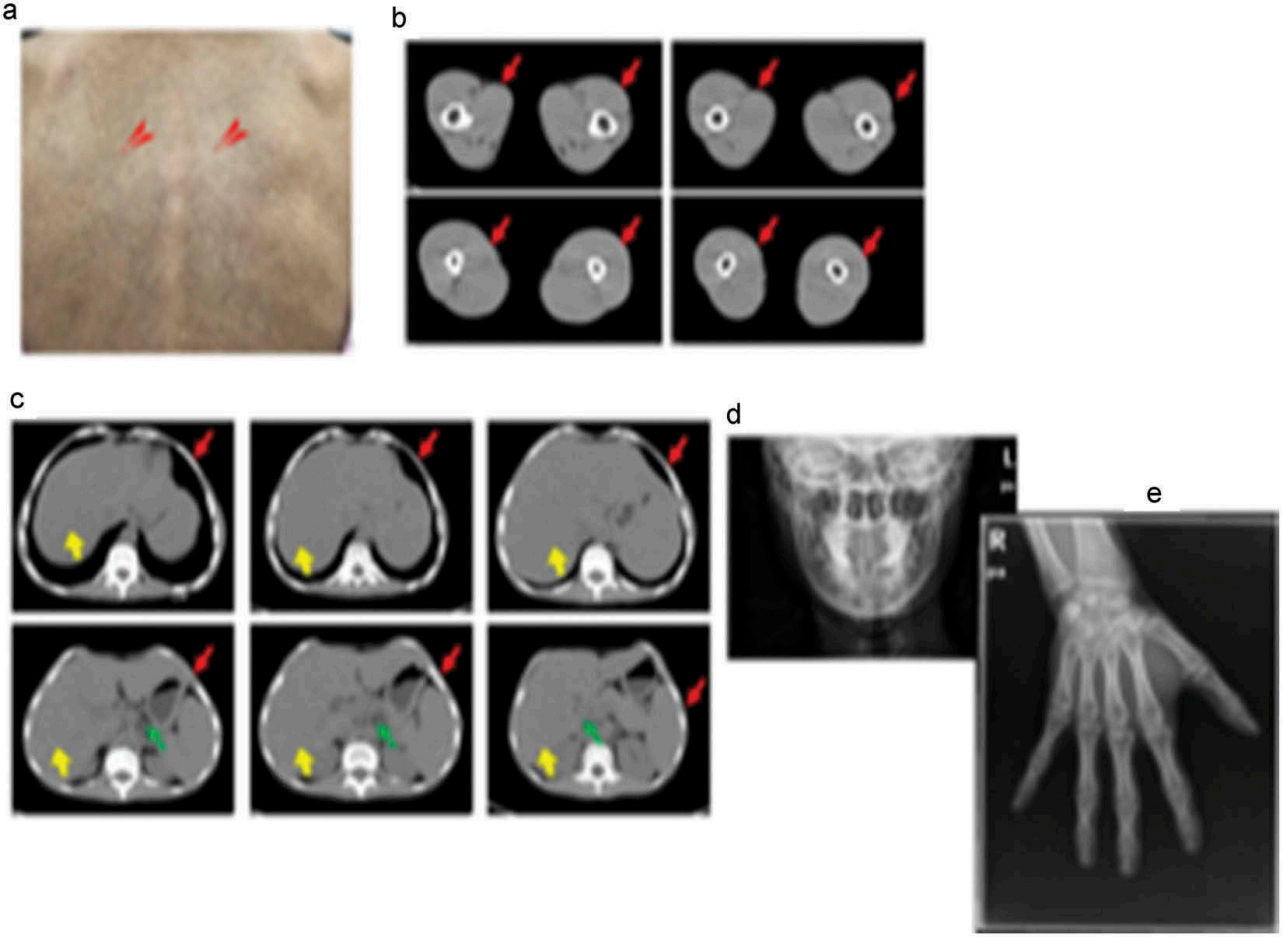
Clinical characteristics of laminopathy in this patient. (a). Mottled hyper-and hypo-pigmentation of the back skin (red arrows). (b). Loss of thigh subcutaneous fat as revealed by computed tomography (red arrows). (c). Liver hypertrophy (yellow arrows) and loss of abdominal subcutaneous (red arrows) and visceral fat (green arrows) as revealed by computed tomography. (d). Micrognathia. (e). Absence of finger acro-osteolysis.
